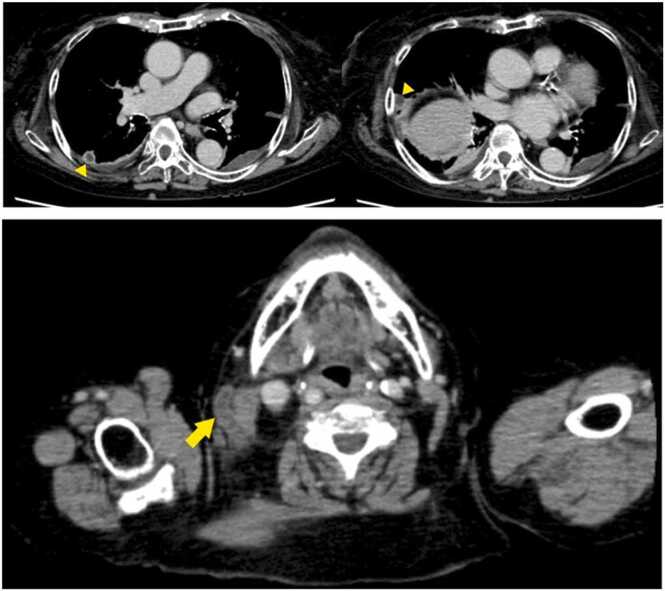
(A) Contrast-enhanced computed tomography scan on day 6 of admission revealed a low-density area inside the multiple lung nodule shadows, and a diagnosis of multiple lung abscesses was made (yellow arrow heads). (B) There was isolated right external jugular vein thrombosis (yellow arrow). (For interpretation of the references to colour in this figure, the reader is referred to the web version of this article.)
